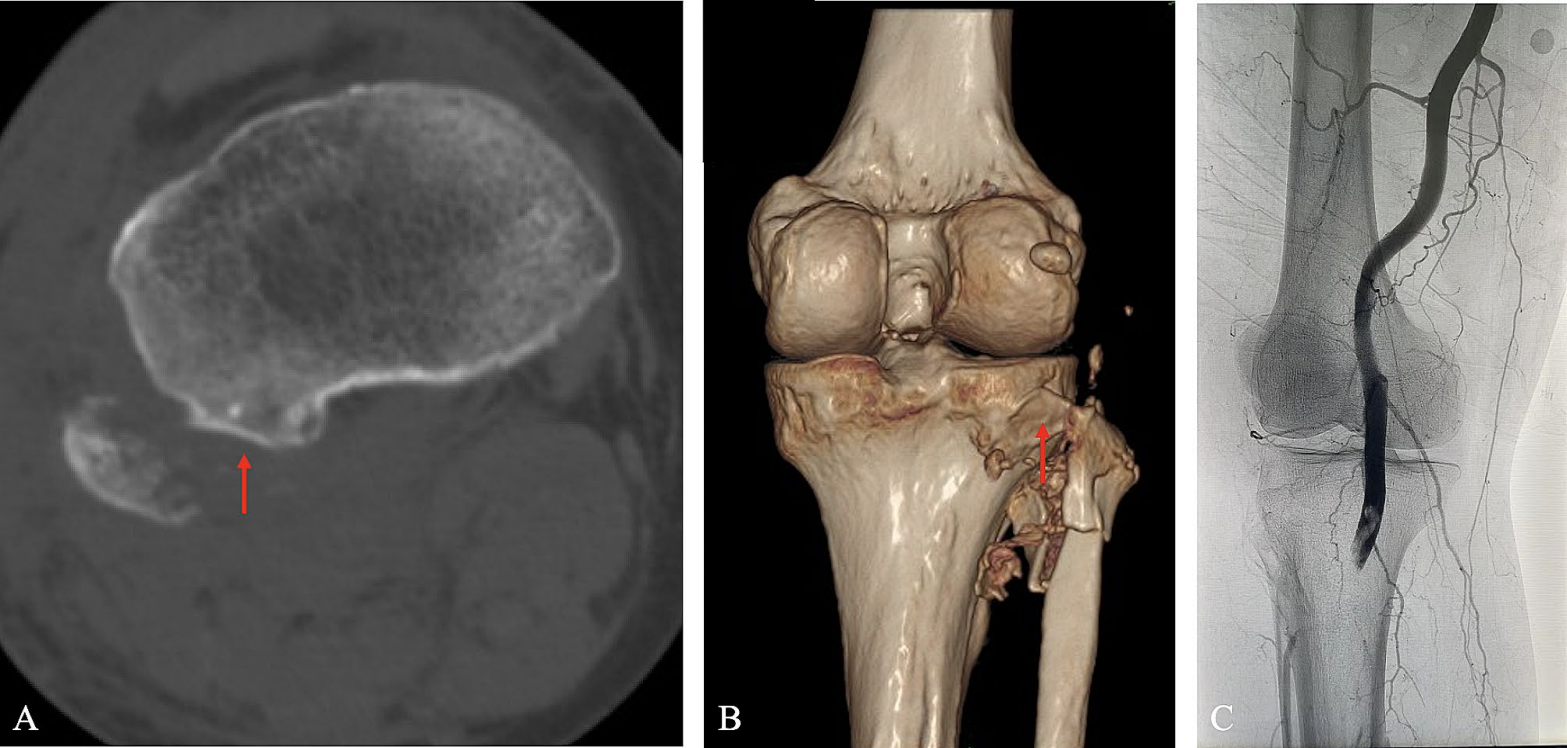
Traumatic proximal tibiofibular fracture and dislocation (anterolateral dislocation). Axial CT (A) and 3D reconstruction (B) imaging shows anterolateral dislocation of fractured fibular head (red arrow indicates the tibial articular surface of proximal tibiofibular joint). Angiography suggests popliteal artery injury (C)
